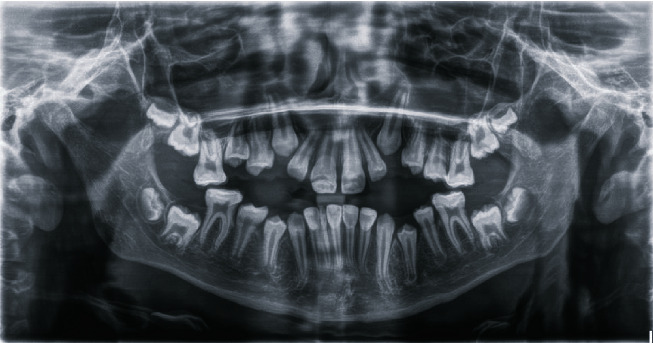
Postoperative panoramic radiographic view.
